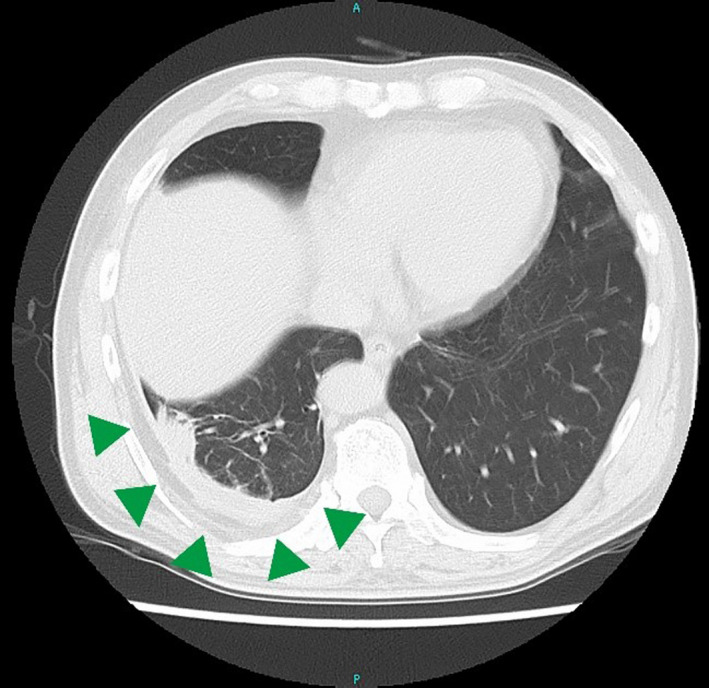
Chest plain computed tomography revealed a high‐density area along the pleura of the right lung base with pleural thickening (arrowheads)
